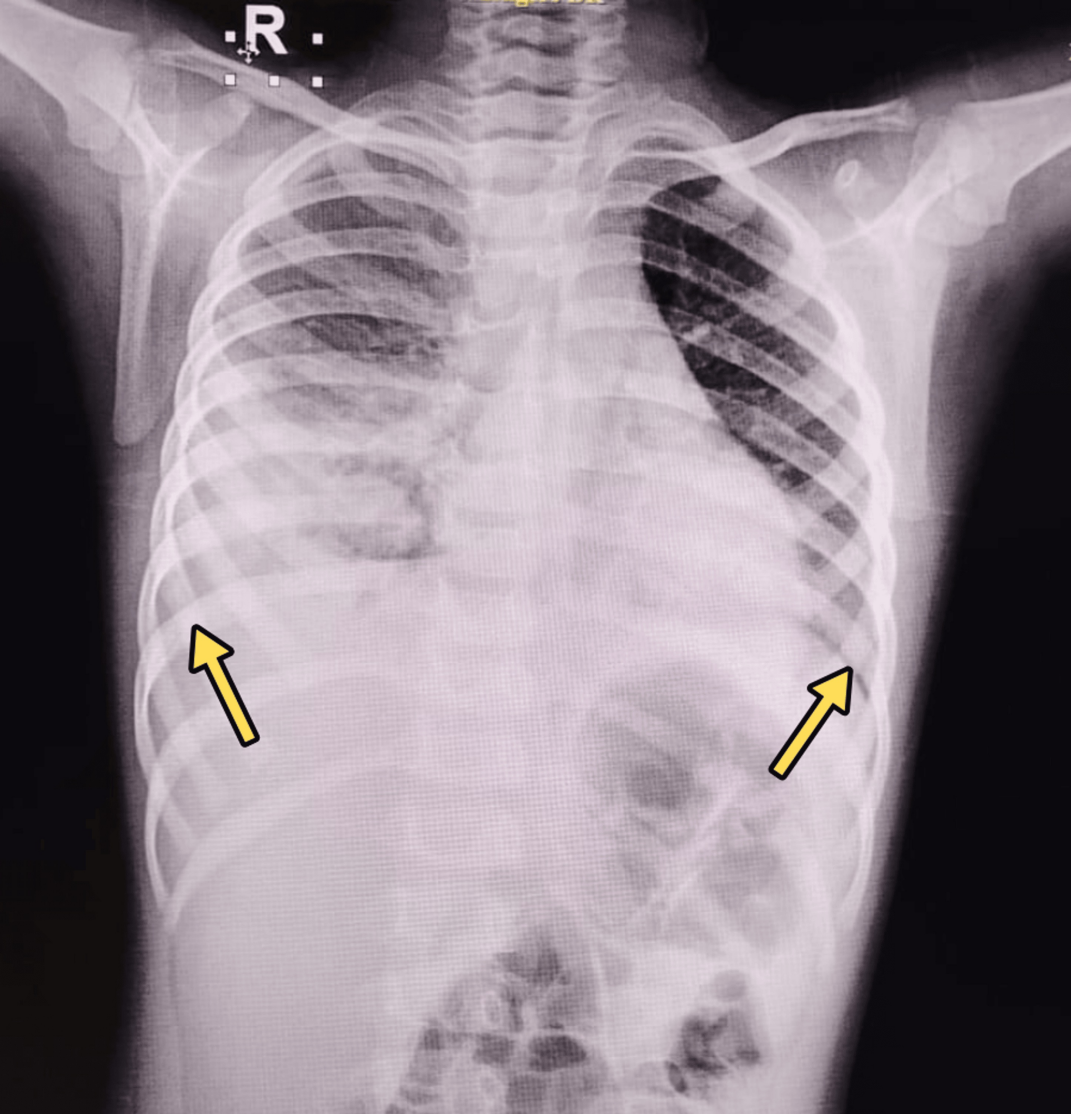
Chest radiograph at admission showed features of pneumonia Chest radiograph demonstrating diffuse consolidation in both lower lobes (arrows).
